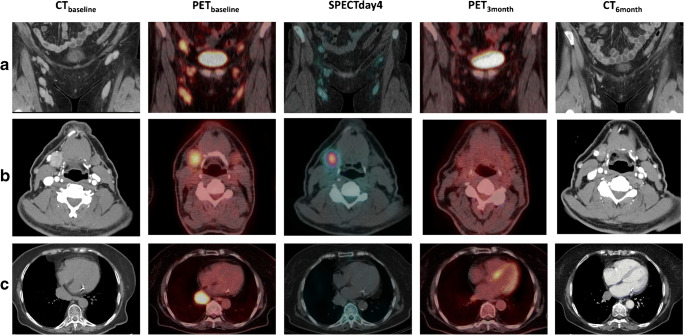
Images obtained at baseline and response evaluation, as well as SPECT/CT images showing the uptake of ^177^Lu-lilotomab satetraxetan at one time point. CT_baseline_, PET_baseline_, SPECT day 4, PET_3months_, and CT_6months_ for a patient 17, b patient 21, and c patient 16
